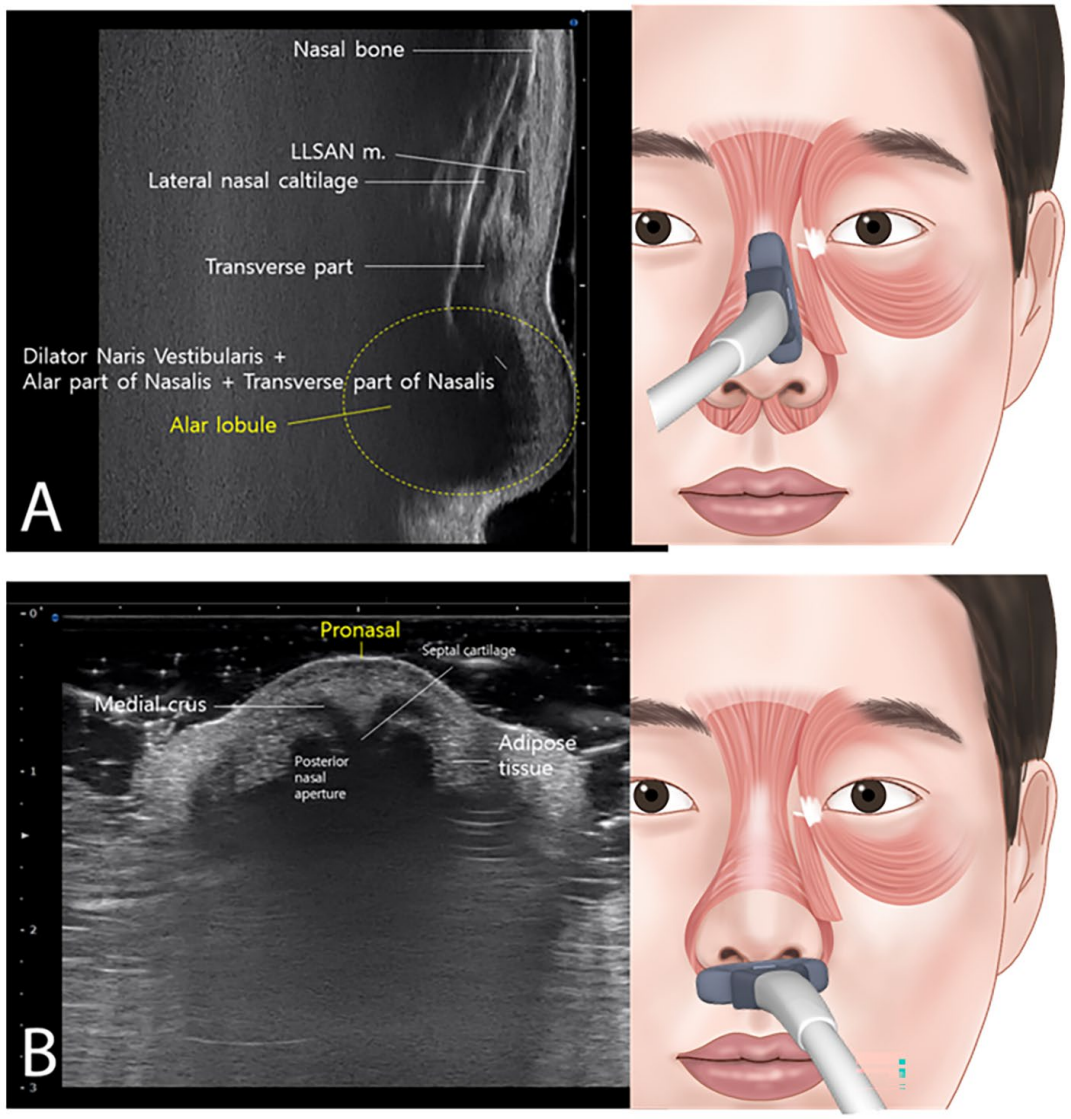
Ultrasound observations during probe placement in specific nasal areas. In panel A, the probe placed diagonally and laterally for observing the transverse part of the nasalis reveals a convergence of the dilator naris vestibularis (DNV), ala part of nasalis, and transverse part of nasalis at the lateral section of the alar lobule. The resulting hypoechoic image displays the presence of the lateral nasal cartilage and associated muscles. In panel B, the probe positioned on the pronasal area provides a view of the medial crus along with the septal cartilage, depicted in the hypoechoic image (B mode, 18‐MHz linear transducer).
